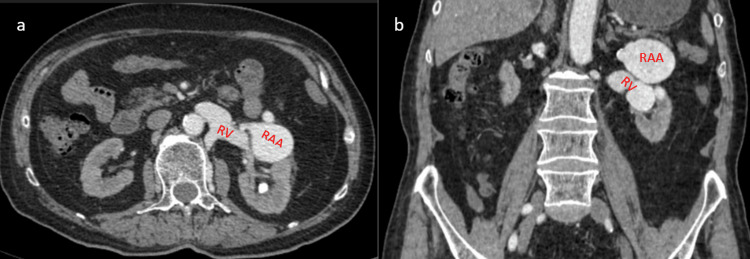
a) The axial plane of computed tomography angiography (CTA) shows a left renal artery aneurysm (RAA) measuring 54x42mm in relation to the left renal vein (RV). b) The coronal plane of the CTA depicts the dilation of the RV in the arterial phase, indicating the arteriovenous fistula (RAVF).
